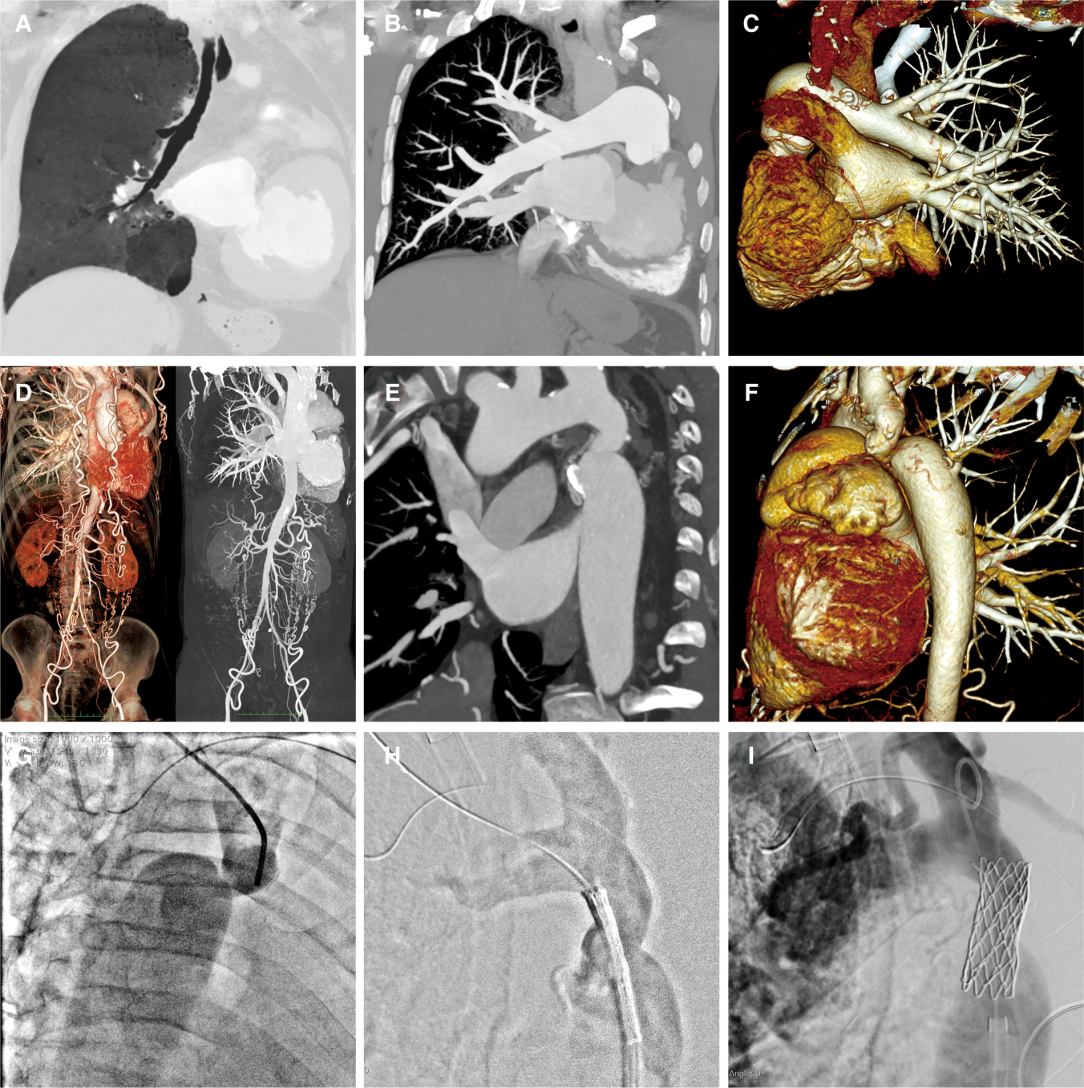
A-C: Pulmonary artery CTA reveals the absence of the left lung structure, with no display of the left pulmonary artery and its branches. The right lung structure and pulmonary artery show no filling defect, and the branches of each lobe segment artery are well displayed with compensatory dilatation. D-F: Aortic CTA shows localized narrowing at the beginning of the descending aorta and branches are formed at the distal ends of the subclavian artery, intercostal arteries, intrathoracic arteries, and both iliac arteries. G-I: Aortic angiography assesses the degree of narrowing, locates the position of the balloon-expandable covered stent, and evaluates the post-release effects.
